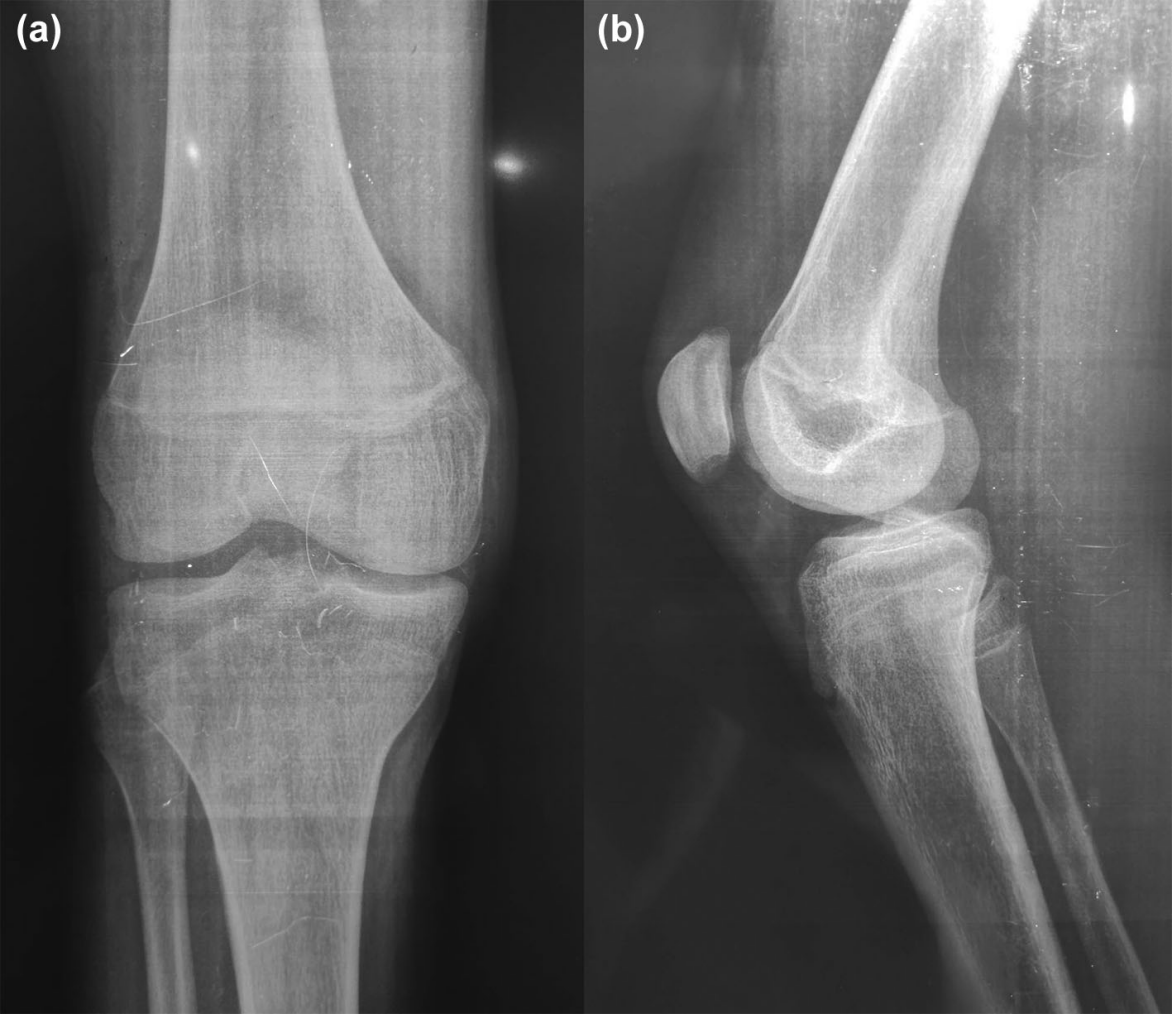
A radiograph of the right knee of a 17-year-old boy showing no detectable abnormalities.
